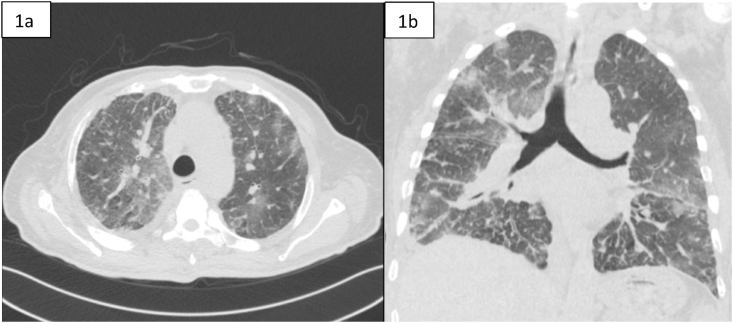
a & 1b: CT chest showing bilateral diffuse reticulonodular ground glass changes but 1a no obvious EM 1b.
